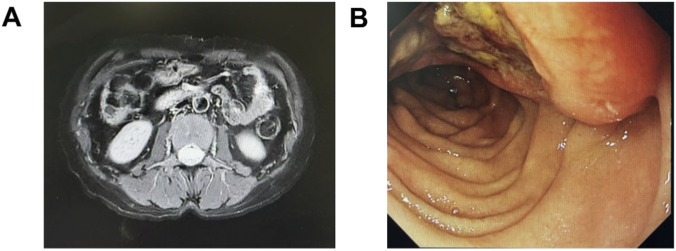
General condition of the patient. (A) Magnetic resonance imaging (MRI); (B) Endoscopic image of the descending part of the duodenum.
